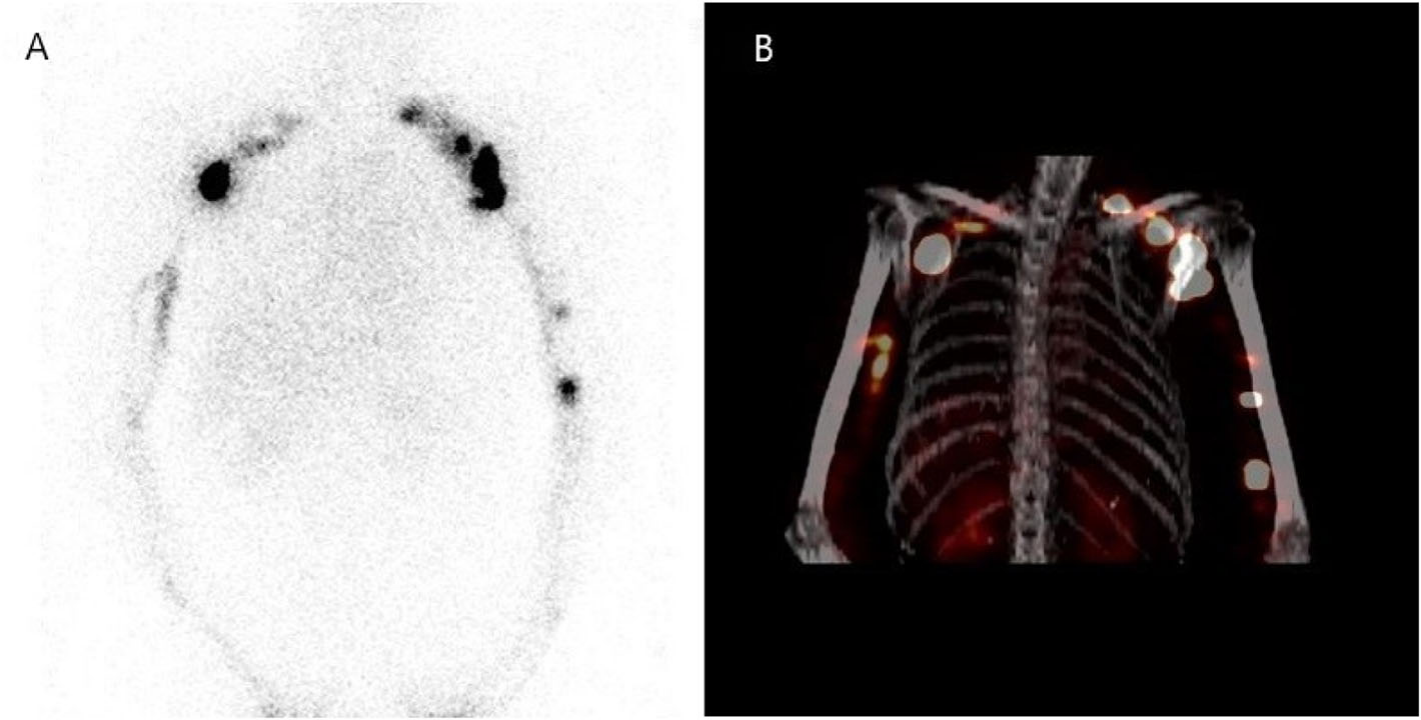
Axillary and clavicular lymph nodes were visualized with planar scintigraphy (a) and SPECT/CT (b) imaging
